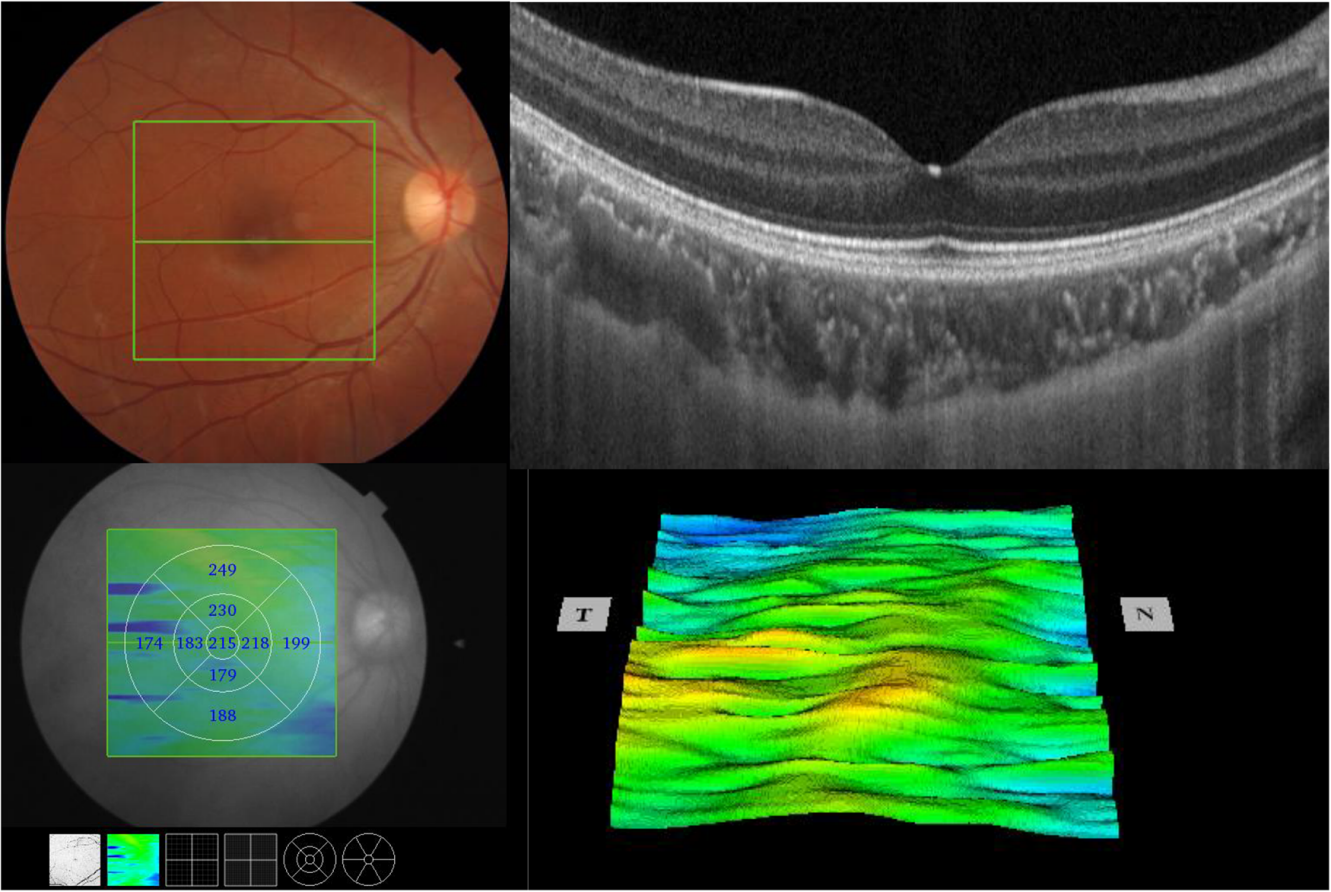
Quantification of choroidal thickness (CT) in macular regions by swept-source optical coherence tomography. Each region's CT was presented using the ETDRS nine-pattern grid, including the central, inner superior, inner nasal, inner inferior, inner temporal, outer superior, outer nasal, outer inferior, and outer temporal fields.
